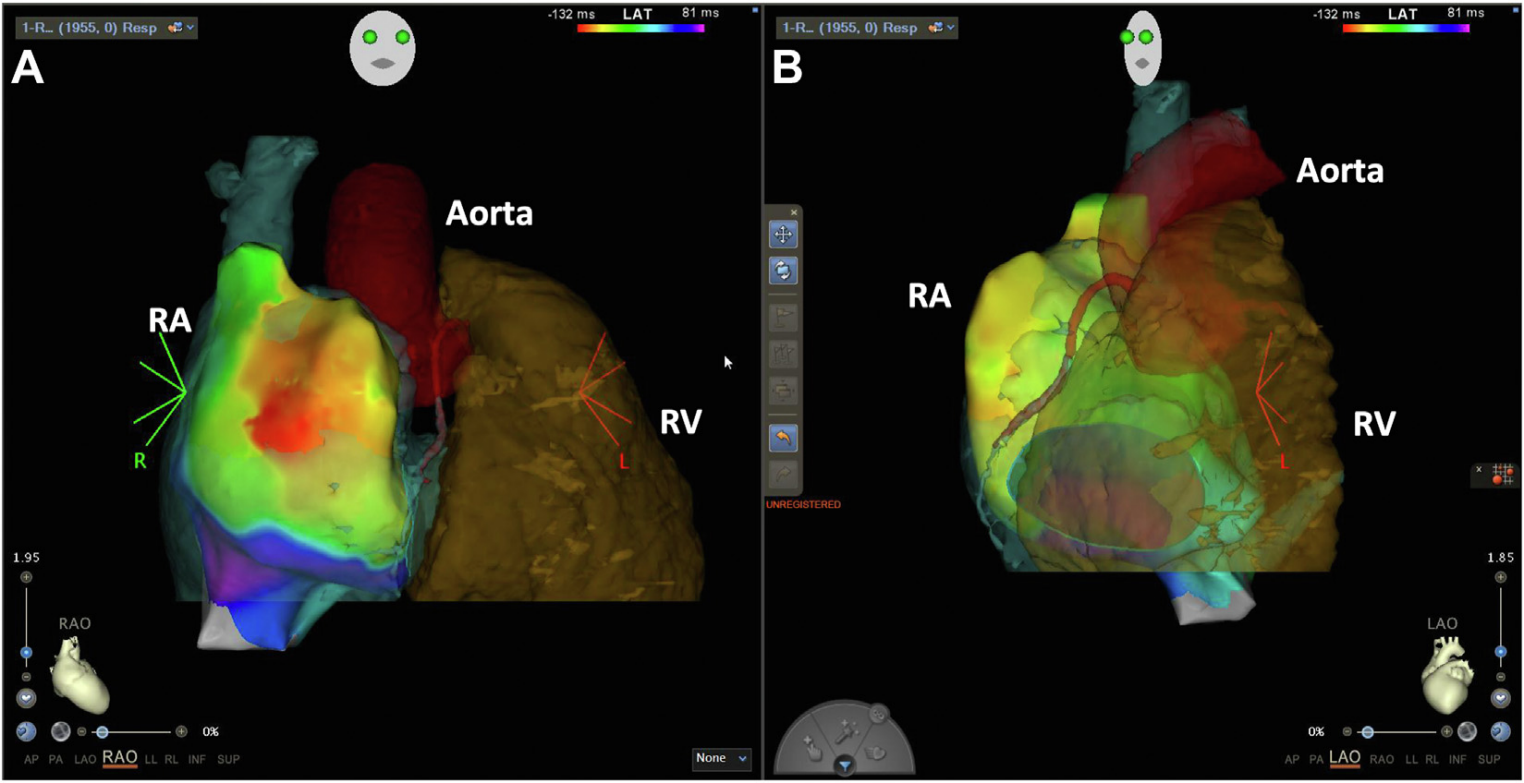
Activation map of sinus rhythm merged with cardiac computed tomography (CT) scan. A and B were the right anterior oblique (RAO) and left anterior oblique (LAO) views, respectively, showing the earliest sinus activation merged with preprocedural contrast CT scan of the heart. RA = right atrium; RV = right ventricle.
